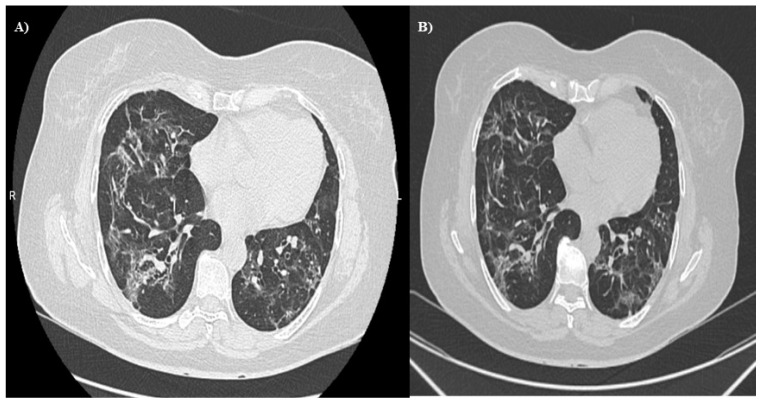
CT scan showing abnormalities in the lungs of one patient following severe COVID-19 infection. (A) Patient is 71 years old, 1-year follow-up post-COVID-19. Bilateral cobblestone lung images predominantly in the upper lobes and the upper segments of the lower lung lobes are associated with subpleural dense linear images. (B) Same patient as Figure 1A. Two-year follow-up post-COVID-19. Pulmonary images of cobblestone pattern in the upper lobes and the upper segments of the lower lobes are associated with images of traction bronchiectasis. Septal thickening in the lower lobes is associated with subpleural dense linear images.
